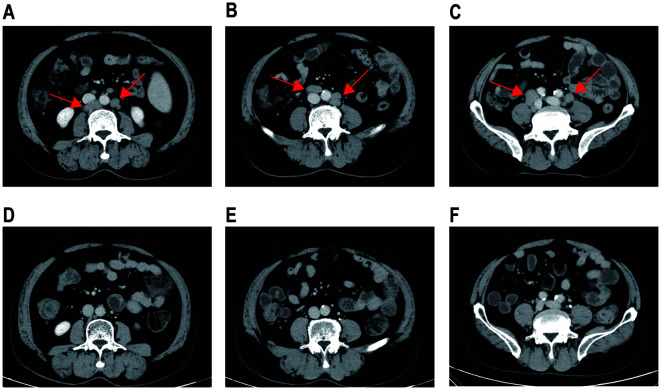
CT images before and after CAR-T therapy. CT scans following two cycles of daratumumab in combination with GemOx showed no treatment response (A–C). One month after the transfusion of CD19 and CD22 CAR-T cells, the patient achieved a complete response (D–F).
